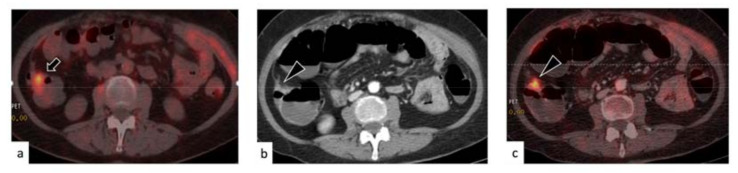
Example of clarification of a paracolic finding with positron emission tomography/contrast enhanced X-ray computed tomography (PET/ceCT). The PET/low dose (ld)CT (a) displayed unspecific-looking intestinal uptake, without a definite CT correlate (arrow). The PET/ceCT (b,c) identified a paracolic nodule, which was suggestive of the presence of active disease (arrowheads). This nodule disappeared after chemotherapy and therefore was confirmed as a true positive finding.
